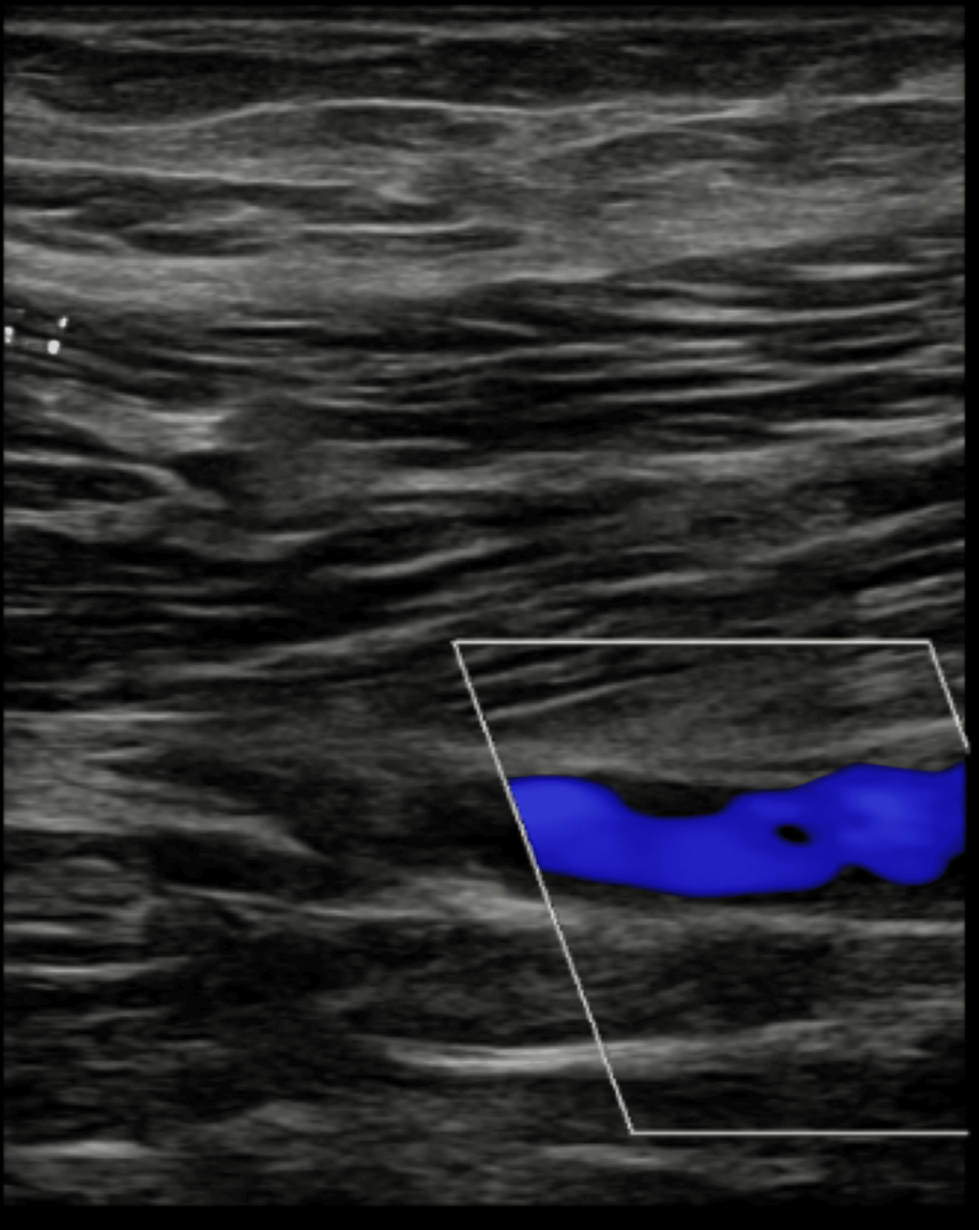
Shows a USS image of the left distal superficial femoral vein where a chronic DVT was visualized. USS: ultrasonography scan; DVT: deep venous thrombosis
